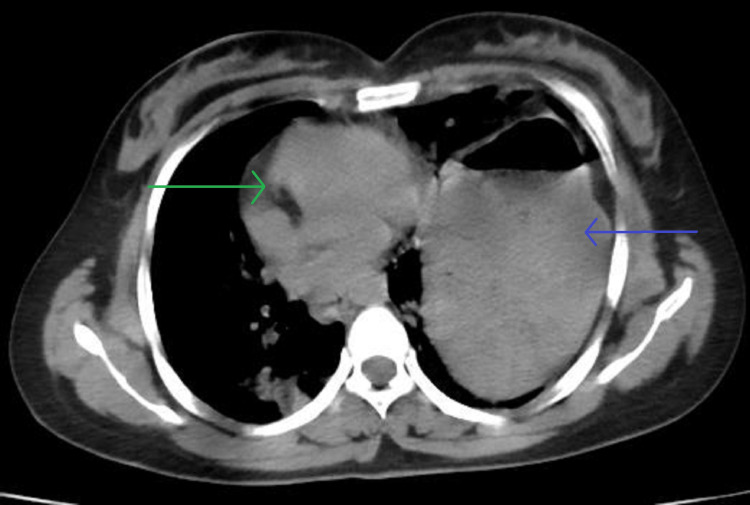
CT Thorax The blue arrow shows the herniated stomach in the left hemithorax, and the green arrow shows the mediastinal shift.
